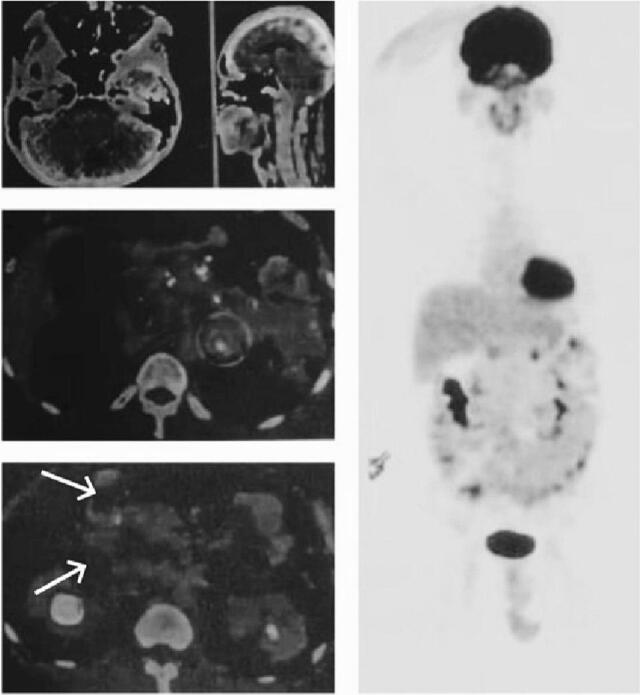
PET/CT scan demonstrated multiple hypodense nodules/cystic (white arrow) with multiple calcifications covering the entire pancreas.
